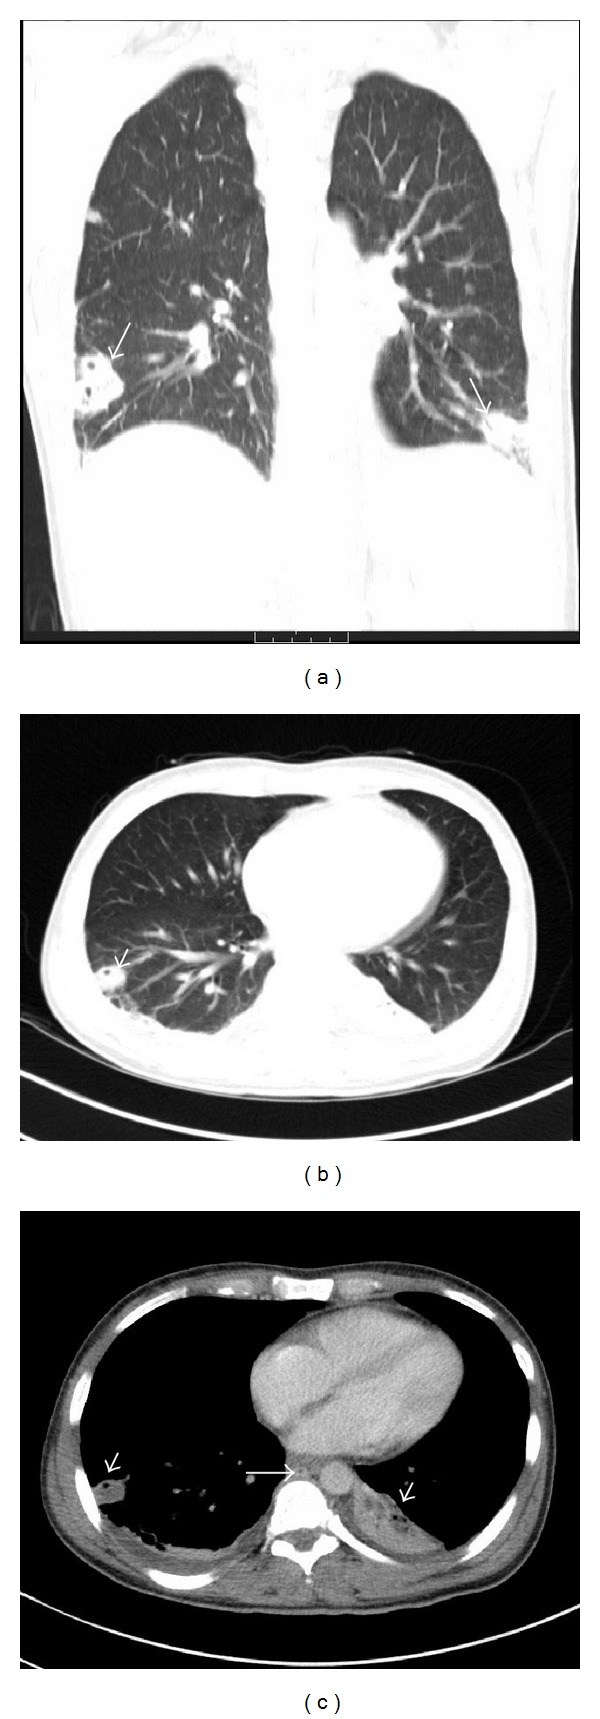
Computed tomography of the chest (panel (a): coronal view—lung window): multiple pulmonary cavitary lung lesions due to septic pulmonary emboli (arrows). Panel (b) (axial view—lung window) and panel (c) (axial view—mediastinal window) show multiple cavitary lung lesions due to septic pulmonary emboli (arrow heads). Filling defect is noted in the azygos vein suggestive of venous thrombus (arrow in panel (c)). There is associated mild pleural effusion bilaterally.
